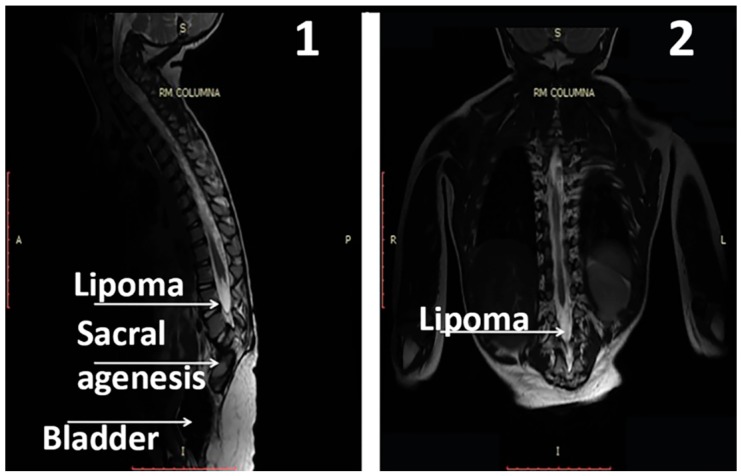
MRI performed at age 3-years old. (1) and (2) show sagittal and antero-posterior images (respectively) of the spinal cord. In 1, sacral agenesis and the hypoplastic L3 vertebra can be clearly seen (white arrow). In both images, a small lipoma can be seen in the lower part of the conus medullaris. As the images show, the SC was normal in its diameter and morphology, but a small hydrosyringomyelia can be seen. In (2), an asymetry between the right and left hip can be observed. A: anterior; P: posterior; S: superior; I: inferior; R: right side; L: left side.
